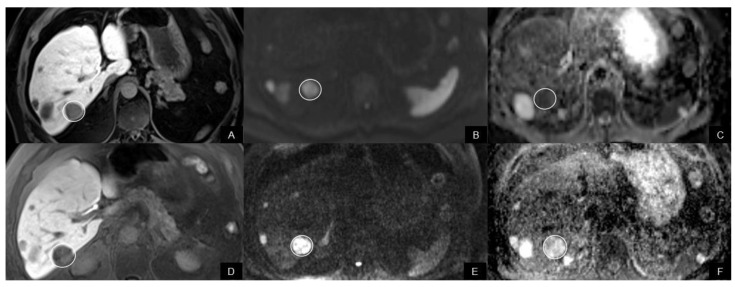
An 74-year-old man with hepatic metastasis of pNET classified as a nonresponder. (A,D) Under everolimus, the metastasis (arrow) increased in size from (A) 25 mm to (D) 29 mm on the axial contrast-enhanced T1W image (liver-specific phase) (B,D). On both (B) pre- and (D) posttherapeutic MRI, the metastasis (arrow) showed restricted diffusion with high signal on DWI image with high b-value. (C) ADCmin value of the metastasis (circle) on pretherapeutic ADC map (E) was 747 × 10^−3^ mm^2^/s and increased to 811 × 10^−3^ mm^2^/s on (F) the post-therapeutic scan.
